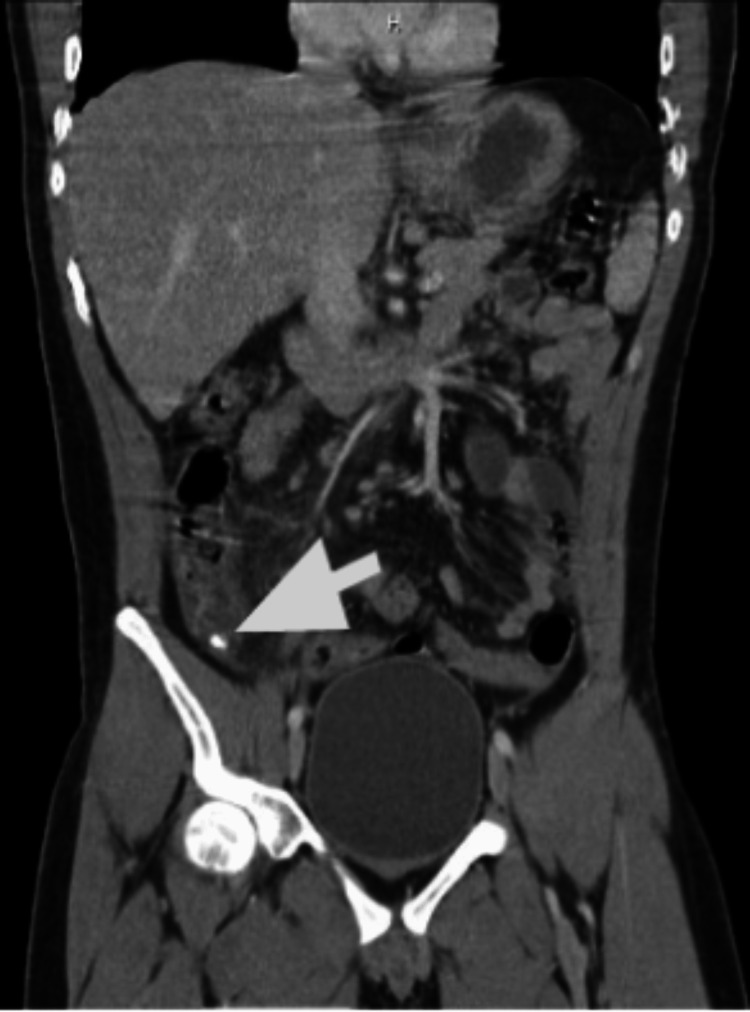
Contrast enhanced CT of the abdomen and pelvis showing acute appendicitis without evidence of perforation
